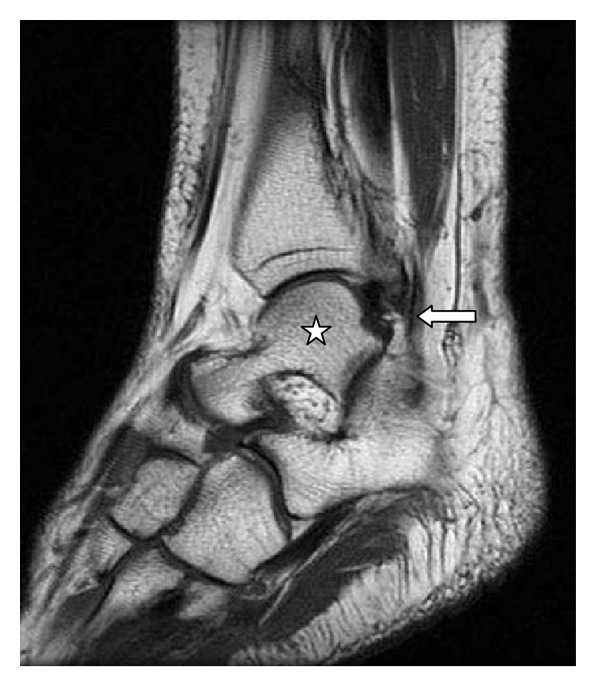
MRI, sagittal T1 23/550 of left ankle. Star: talus bone; arrow: fibularis quartus muscle.
